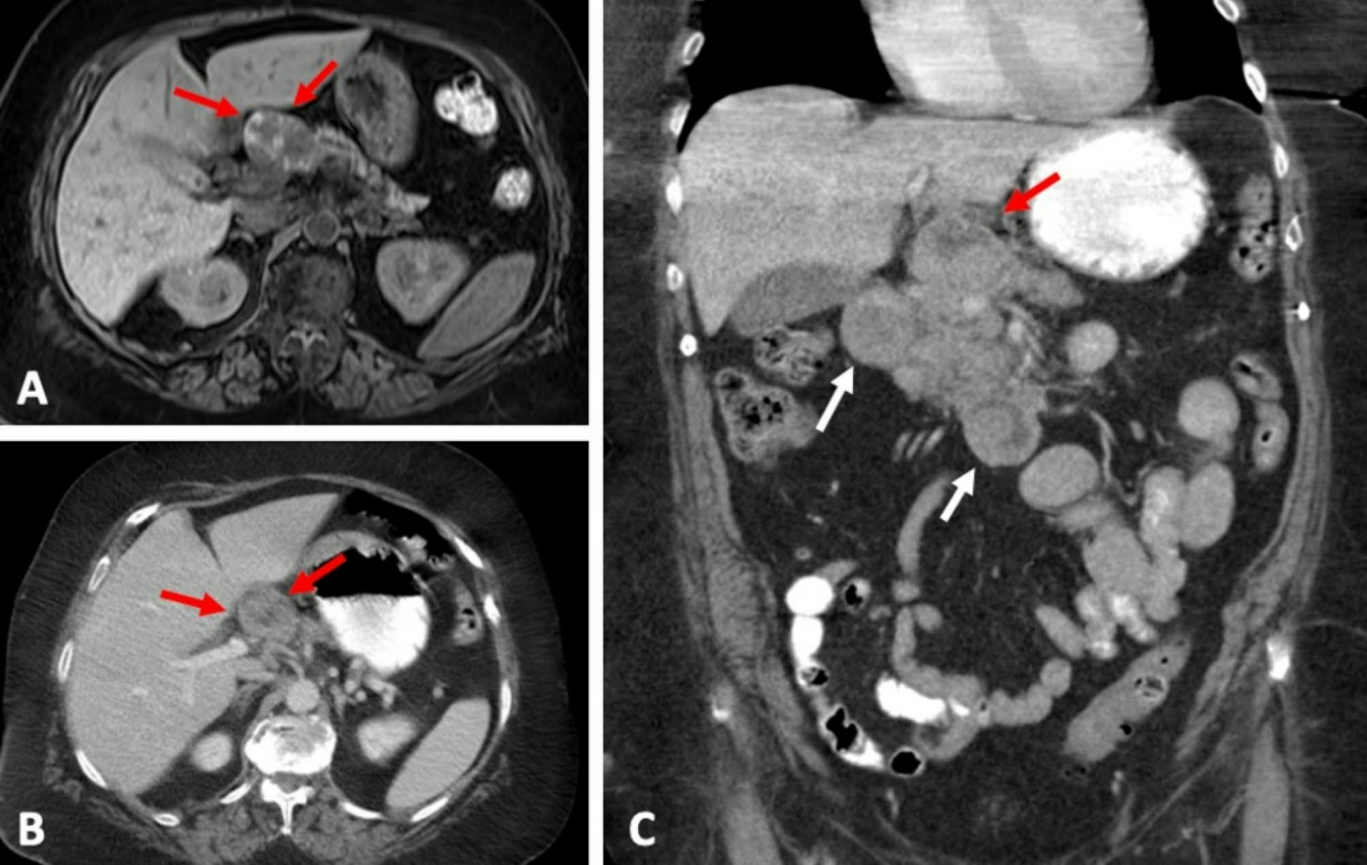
78-year-old female with acinar cell carcinoma in the pancreatic head (red arrows) demonstrating hyperintensity on axial T1-weighted MR image (A) and hypoattenuating components on CT (B, C). Locoregional metastasis to the peripancreatic lymph nodes (C) (white arrows) was present at the time of diagnosis. The patient died 248 days after diagnosis
